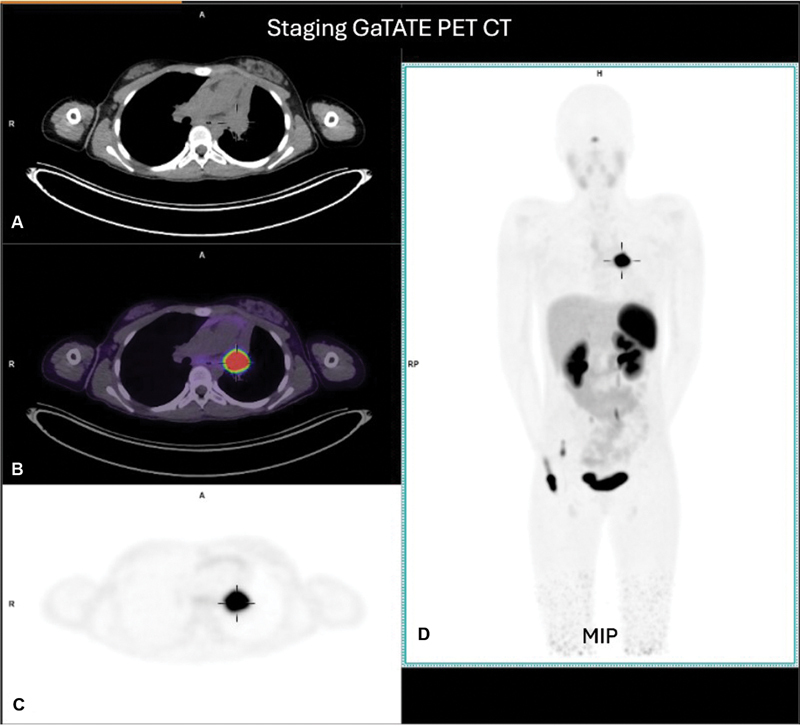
Staging GaTATE PET CT scan. Axial slices of the low-dose CT scan (
